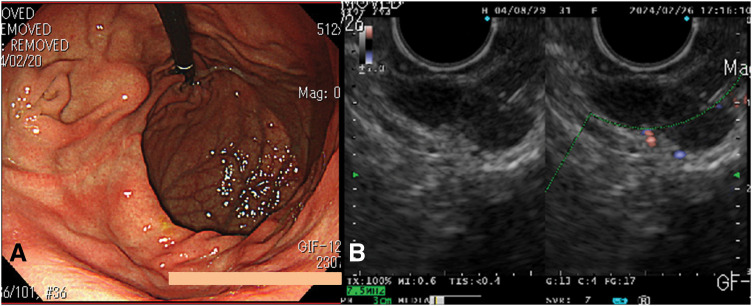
Representative images from abdominal examinations. (A) The upper gastrointestinal endoscopy revealed a submucosal tumor on the lesser curvature wall of the remnant stomach. (B) The endoscopic ultrasound showed 3 hypoechoic masses with a maximum diameter of 16 mm, which were suspected to be submucosal tumors.
